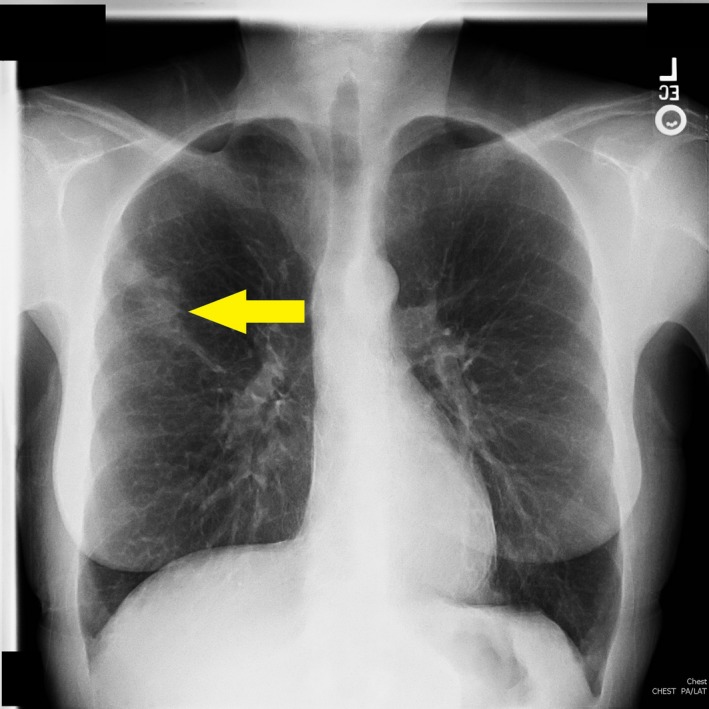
Inflammatory pseudotumor plain film
